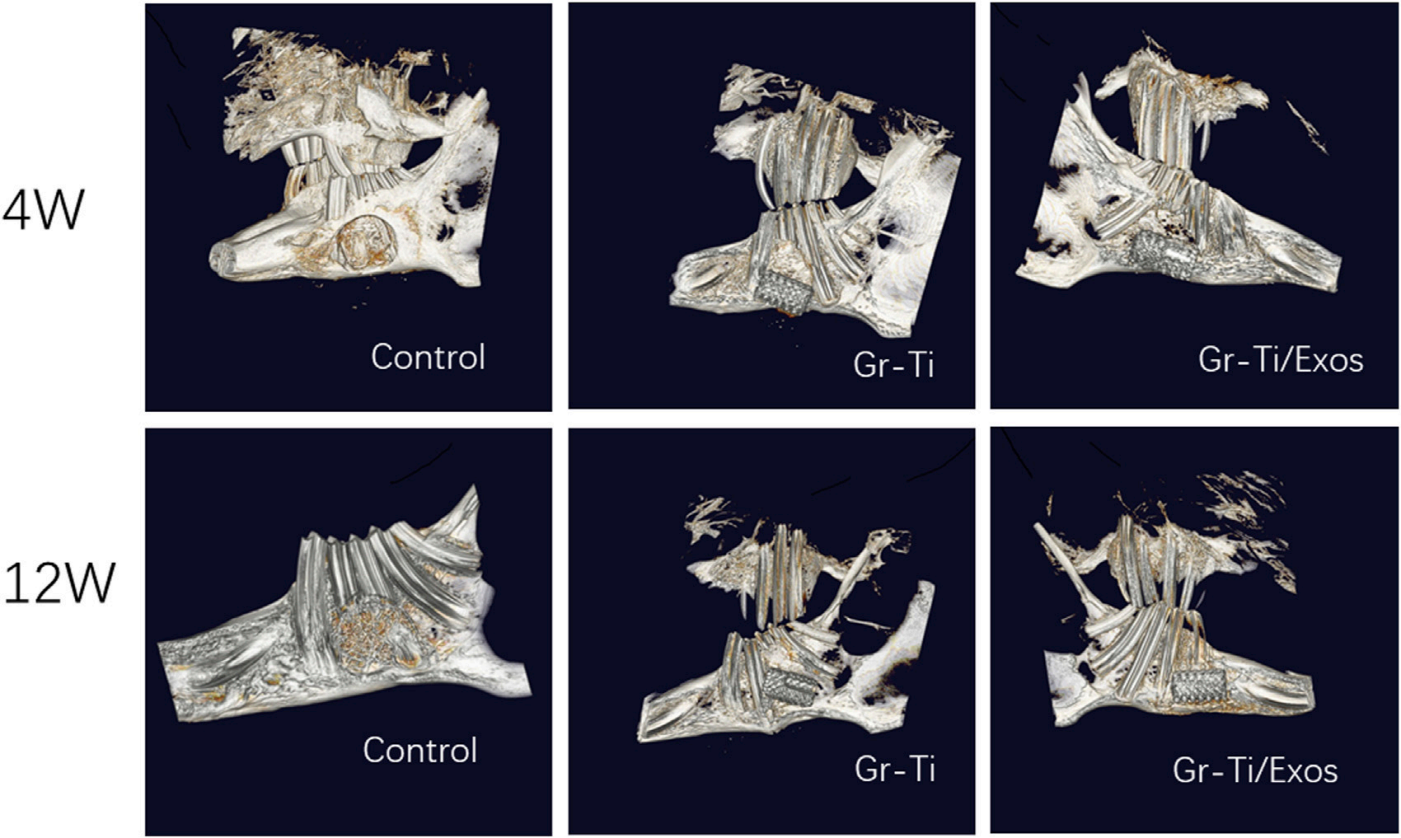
Computerized tomography (CT) images after 4 and 12 weeks.
